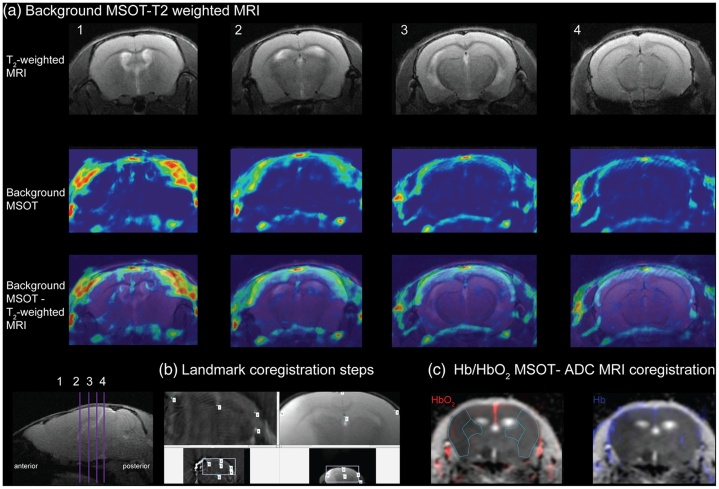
The procedure of MSOT-MRI data coregistration: (a) Coregistration of background MSOT and T2-weighted MRI images in the cross-sectional axial, (b) Landmark coregistration steps, (c) Coregistration result of Hb/HbO_2_ map by MSOT and apparent diffusion coefficient (ADC) map by MRI [85].
